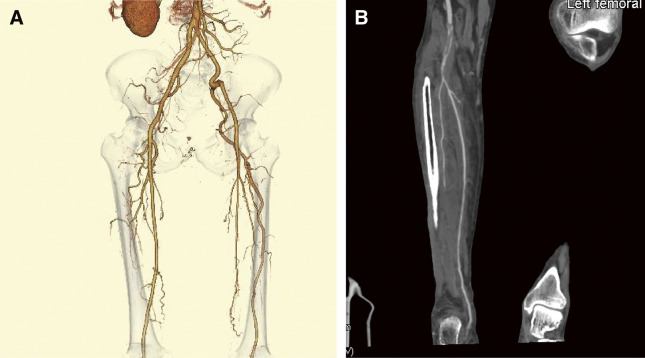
Fig. 2 CT revealed occlusion of the PSA in his left lower extremity. (A) The left PSA originates from the internal iliac artery, passes through the greater sciatic foramen alongside the sciatic nerve, and runs laterally to the insertion of the adductor magnus muscle before continuing as the popliteal artery. The SFA is completely absent. (B) The left PSA was occluded with acute thrombosis in the popliteal artery P2 segment and stenosed with atherosclerosis in the P3 segment. SFA: superficial femoral artery; CT: computed tomography; PSA: persistent sciatic artery
